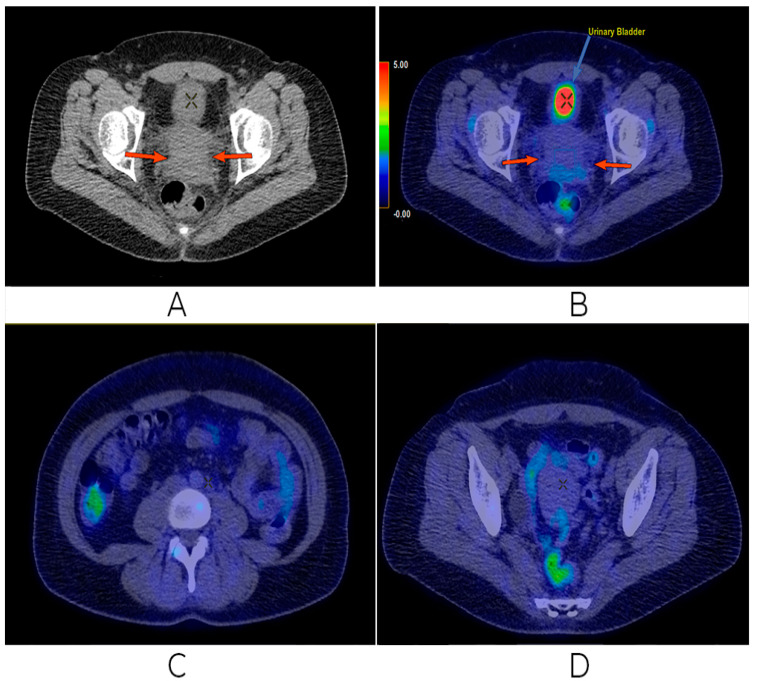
Last FDG PET/CT performed. Complete metabolic remission of the uterine cervical tumor (A,B), as well as the disappearance of the retroperitoneal (C) and left external iliac (D) adenopathies.
